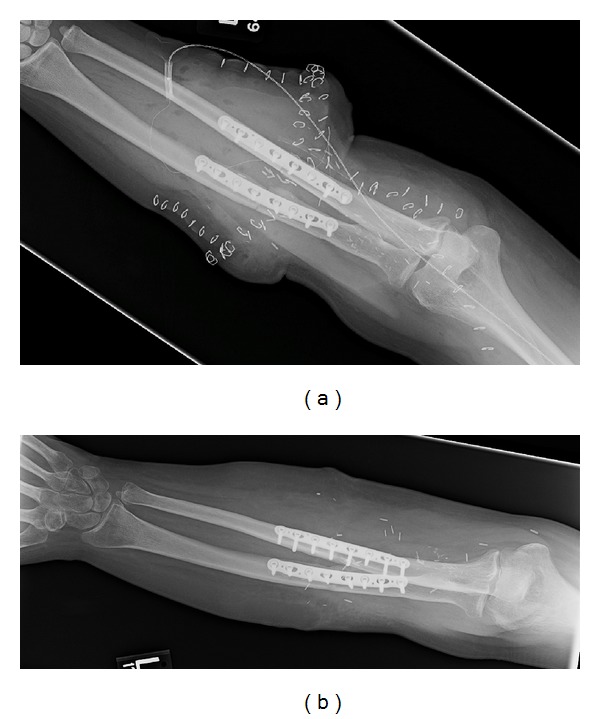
(a) Immediate postsurgical radiograph shows anatomic bony alignment and hardware with extensive soft tissue swelling. (b) One-year follow-up showing decreased swelling and interval osseous healing.
